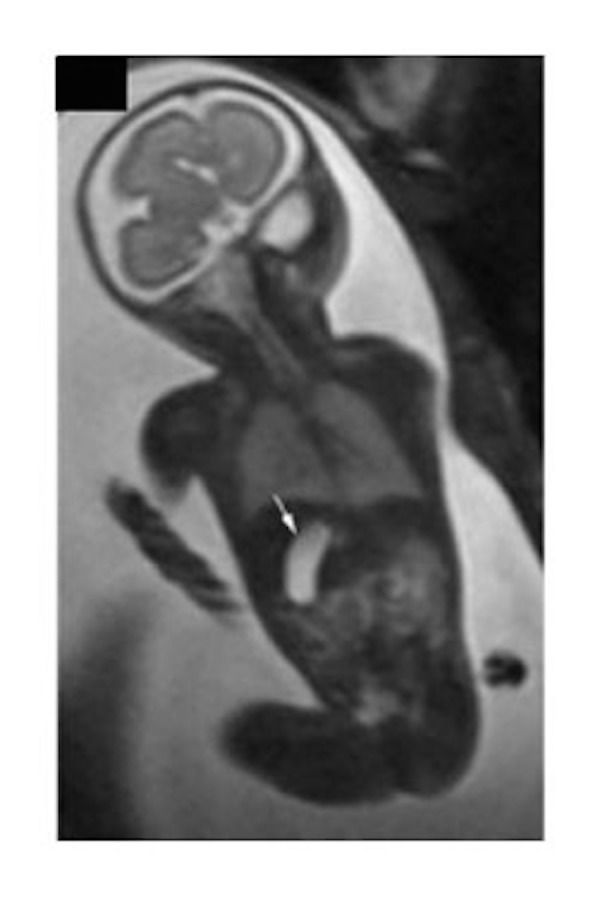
Abnormal abdominal situs T2-weighted HASTE image in the coronal plane demonstrating a right-sided stomach and left-sided liver. Image reprinted without change from Martin, et al. under the creative commons license.
